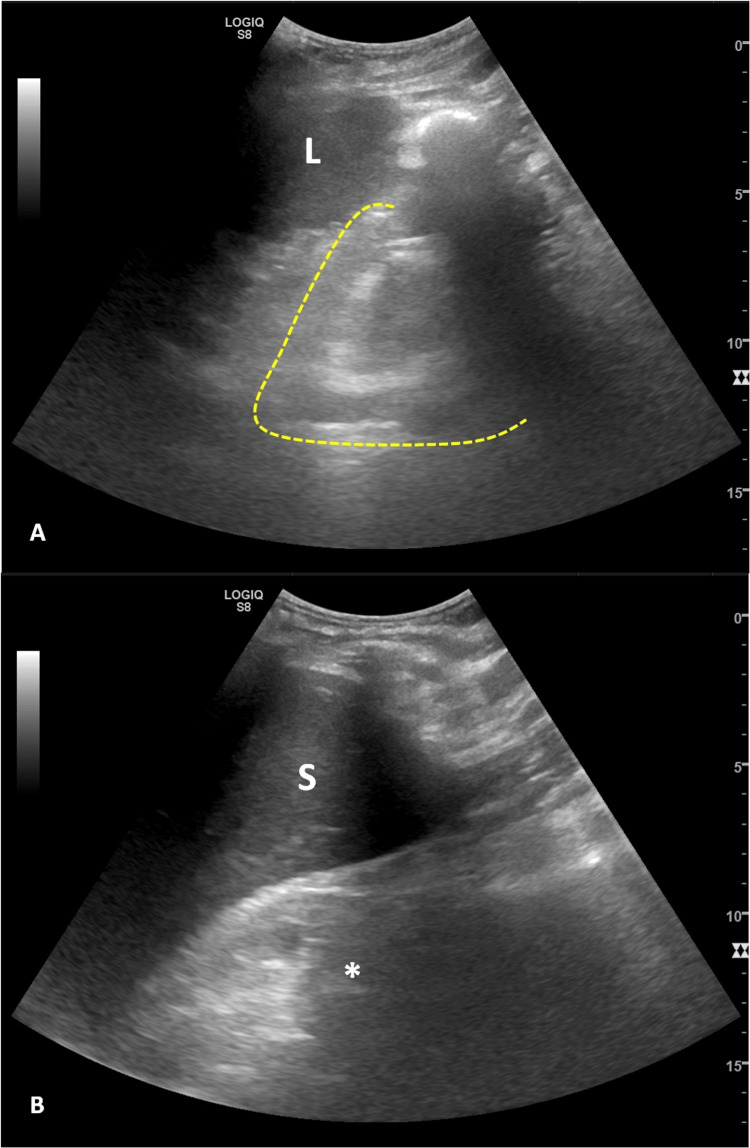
Abdominal and renal ultrasound without apparent abdominal changes and demonstrating a marked reduction in the differentiation of the right kidney (A - yellow dashed line) and absence of the left kidney (B - asterisk). Study limited by gas interposition. L, liver; S, spleen.
